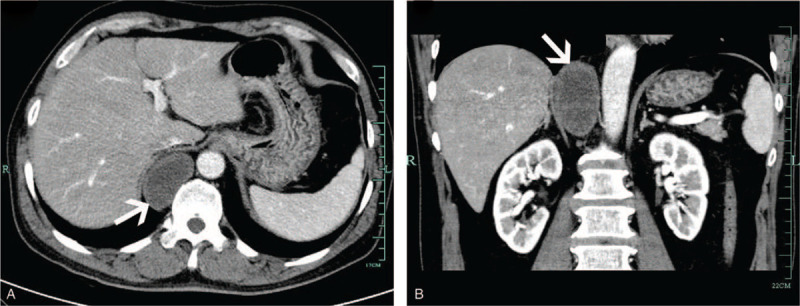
(A) Axial CT image showing the posterior mediastinal mass (white arrows); (B) Coronal CT image showing the posterior mediastinal mass (white arrows).
